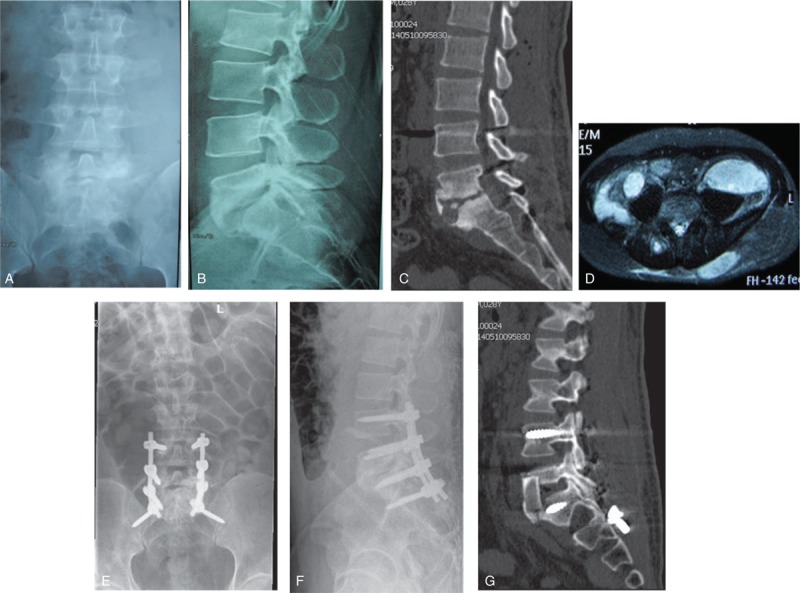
A 29-year-old male patient presented with lumbosacral spinal tuberculosis. (A and B) Preoperative X-ray films of the lumbar spine. (C) Preoperative computed tomography (CT) sagittal films shows significant bone lesion on L3 and L4 vertebral bodies. (D) Preoperative magnetic resonance imaging shows huge prevertebral and abdominal cold abscess. (E and F) The patient underwent combined anterior debridement, strut bone grafting with posterior instrumentation. (G) The CT sagittal construction film of lumbosacral spine at 1 month postoperatively.
